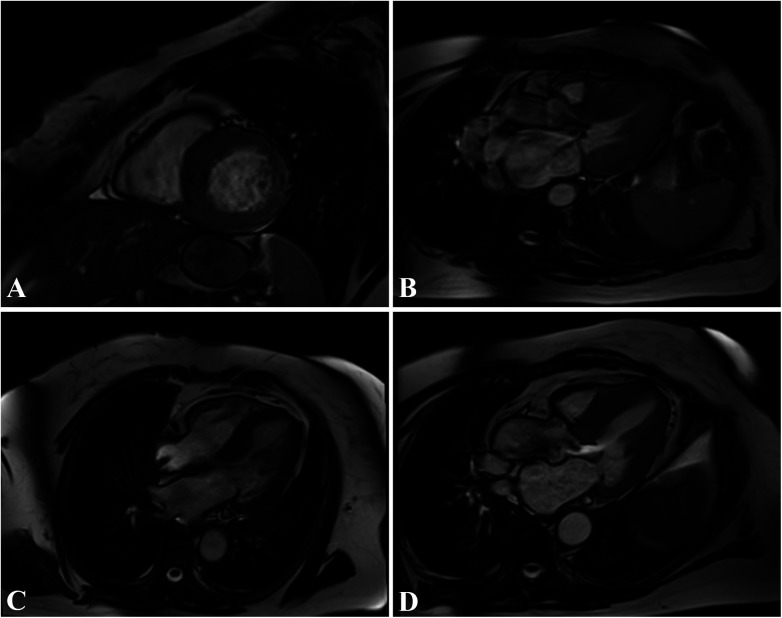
58-year-old patient with asymmetric apical hypertrophy. Four-chamber balanced-steady state free precession (b-SSFP) MRI (A) shows asymmetric apical thickening of the left ventricle with maximum myocardial thickness of 22 mm. PSIR sequence highlights patchy intramyocardial LGE in four-chamber (B) and two-chamber (C) T1 native Mapping (D) demonstrates increased intramyocardial value in the left ventricular walls.
